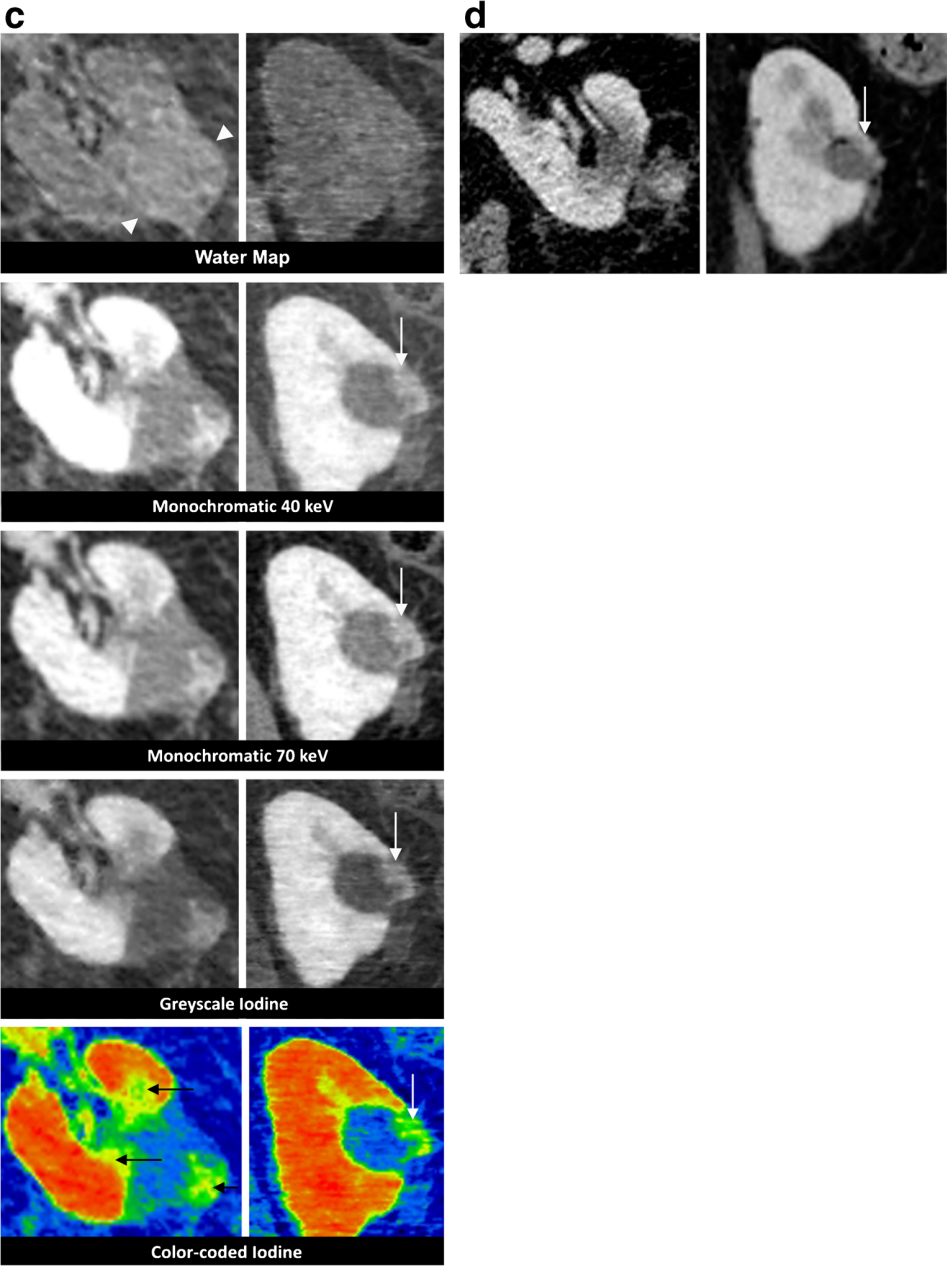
Renal cell carcinoma. An ultrasound reveals a renal mass in a 72-year-old male. a Venous phase DECT confirms a lesion in the left kidney. On the water map images, the lesion is exophytic and isodense compared to the kidney parenchyma (*arrows*). The synthesised monochromatic 40-keV greyscale-coded and colour-coded iodine images show a hypovascular lesion compared to the kidney parenchyma. A biopsy demonstrates a renal clear cell carcinoma. b On the iodine-coded image (left), three ROIs are selected: L1 (red) in the RCC; L2 (blue) in the renal vein; L3 (yellow) in the unaffected kidney parenchyma. The estimated amounts of iodine in the ROIs are presented in the right lower corner (mean value and standard deviation in 100 μg/cm^3^). On the spectral HU curve (right), the renal vein (L2) and RCC (L1) represent overlapping curves, suggesting similar iodine content. The normal kidney parenchyma (L3) has a markedly higher curve in the lower keV range, confirming a higher iodine uptake. c Axial (left column) and coronal (right column) reconstructions from a contrast-enhanced venous phase DECT, 24 h post-RF ablation. The water map images show very poor hyperattenuation in the ablated zone (*arrowheads*). The contrast difference between the ablation zone and the kidney parenchyma is well demonstrated on the synthesised monochromatic 40- and 70-keV images. The colour-coded iodine images show well-depicted areas of intermediate density on the axial reconstructions (*black arrows*) surrounding the ablation zone. The axial images of the synthesised monochromatic reconstructions less clearly visualise these regions. The multiplicity suggests a benign post-ablative finding, probably corresponding to transient thermal damage to the bordering kidney parenchyma. No definitive conclusion can be made concerning residual tumour solely relying on these images. However, compared to the pre-ablation imaging, we can assume that the tissue in question is a normal kidney parenchyma. At the outer margin of the ablation zone, a region of intermediate density is depicted. The coronal reconstructions are essential to prove the continuity with regard to the kidney parenchyma (*white arrows*). d On the follow-up CECT 15 months later, the focally enhanced tissue at the lateral side shows no signs of growth (*arrow*), thus confirming the absence of residual tumour
